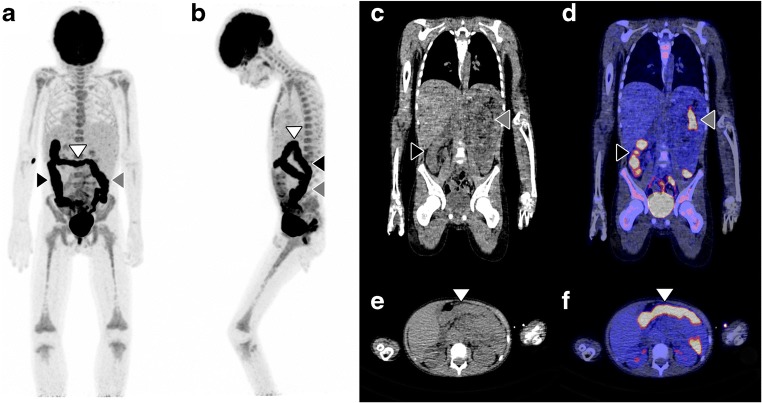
A 9-year-old boy presented at the hospital with anorexia, weight loss, fatigue, and intermittent fever up to 39.5 °C. The patient had experienced these symptoms episodically over the past 18 months, but a definite diagnosis had never been established. On physical examination, the upper abdomen was tender to palpation. Laboratory examinations showed a CRP of 49 mg/L, leukocyte count of 8.0 × 109/L, and erythrocyte sedimentation rate of 46 mm/h. Microbiological urine, blood, and fecal cultures were negative. Abdominal ultrasonography and MRI showed focal steatosis of the liver, but no possible cause of fever. Coronal and sagittal maximum intensity projection FDG-PET (A and B), low-dose CT (C) and fused FDG-PET/CT (D), axial low-dose CT (E), and axial fused FDG-PET/CT (F) showed extensive FDG uptake throughout the entire colon, including the ascending (black arrowheads), transverse (white arrowheads), and descending colon (gray arrowheads). Inflammatory bowel disorder was suspected, and intestinal biopsy established the final diagnosis of Crohn’s disease.
